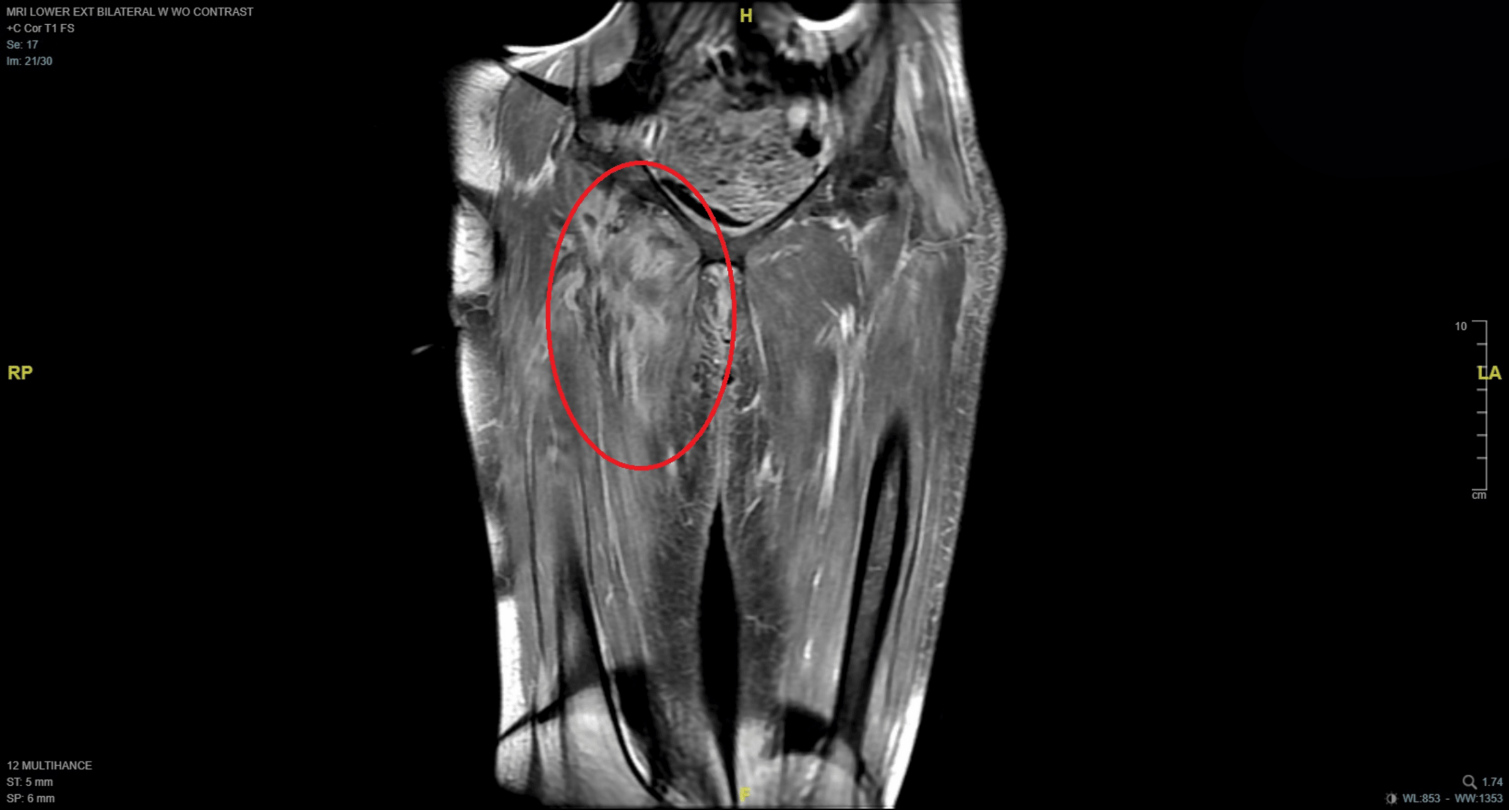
Coronal MRI of both lower extremities with a red circle highlighting the area of hyperintensity in the right lower extremity.
